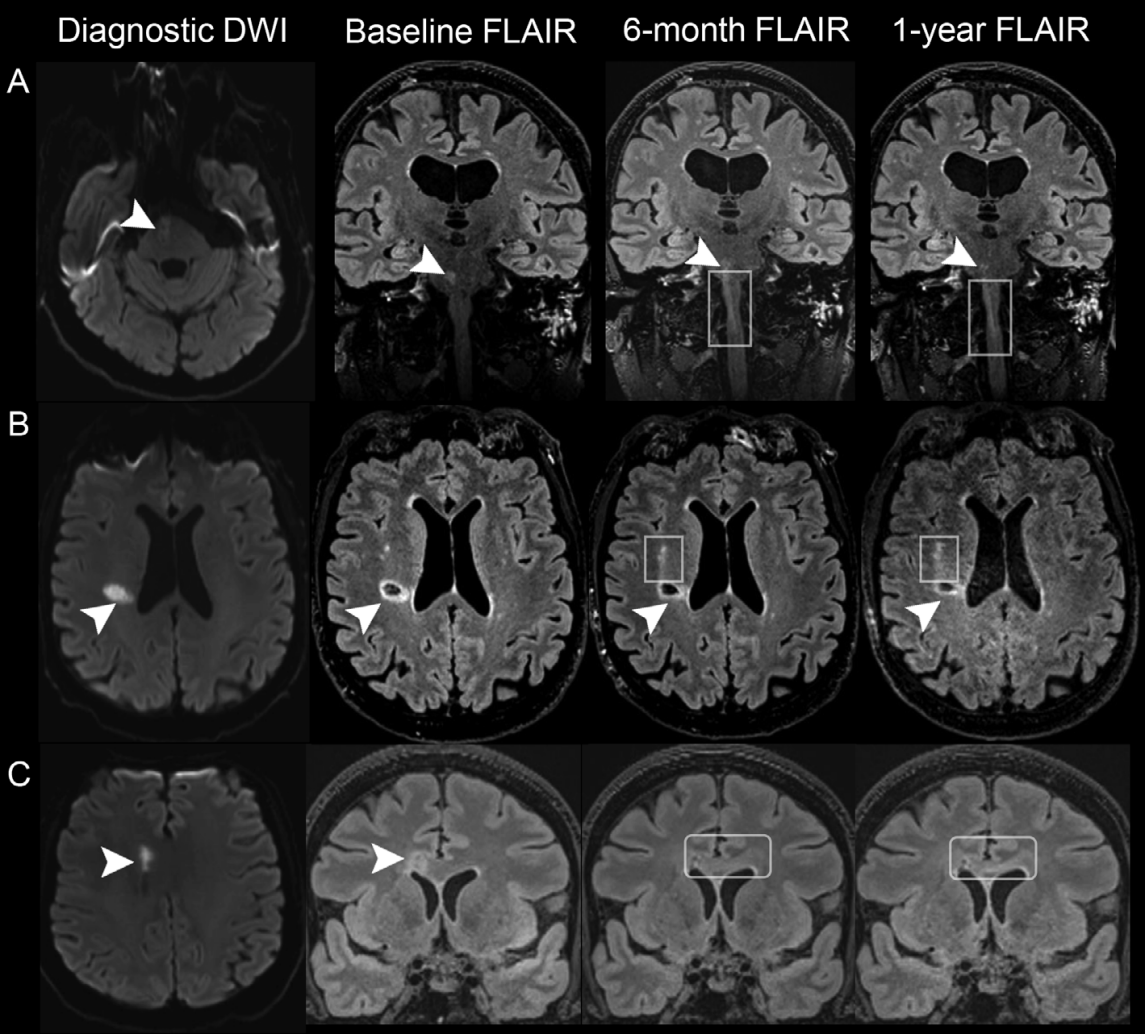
Different types of ‘tracks’ depending on the location of recent small subcortical infarct (RSSI). (Top row, A) In a patient with RSSI in the right pons (arrowheads), a ‘track’ develops and is visible on the 6‐month and 1‐year FLAIR scans as a trajectory descending from the index lesion toward the medulla (squares). (Middle row, B) In a patient with RSSI in the peripheral right coronal radiata (arrowheads), a ‘track’ develops and is visible on the 6‐month and 1‐year FLAIR scans, extending in the anterior intrahemispheric direction (squares). (Bottom row, C) In a patient with RSSI close to the corpus callosum (arrowheads), a ‘track’ develops and is visible on the 6‐month and 1‐year FLAIR scans. The ‘track’ traverses from the right to left hemisphere across the corpus callosum (squares). Note that the visibility of ‘tracks’ increases over time.
